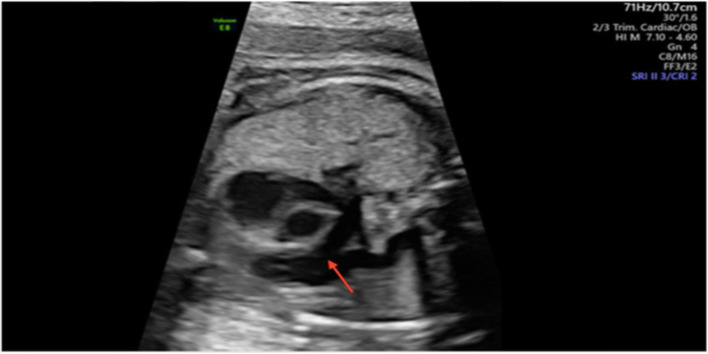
Ultrasound image of the fetal branching pulmonary artery in an extended three vessel view. The red arrow marks the area where the Doppler velocimetry waveform was obtained
